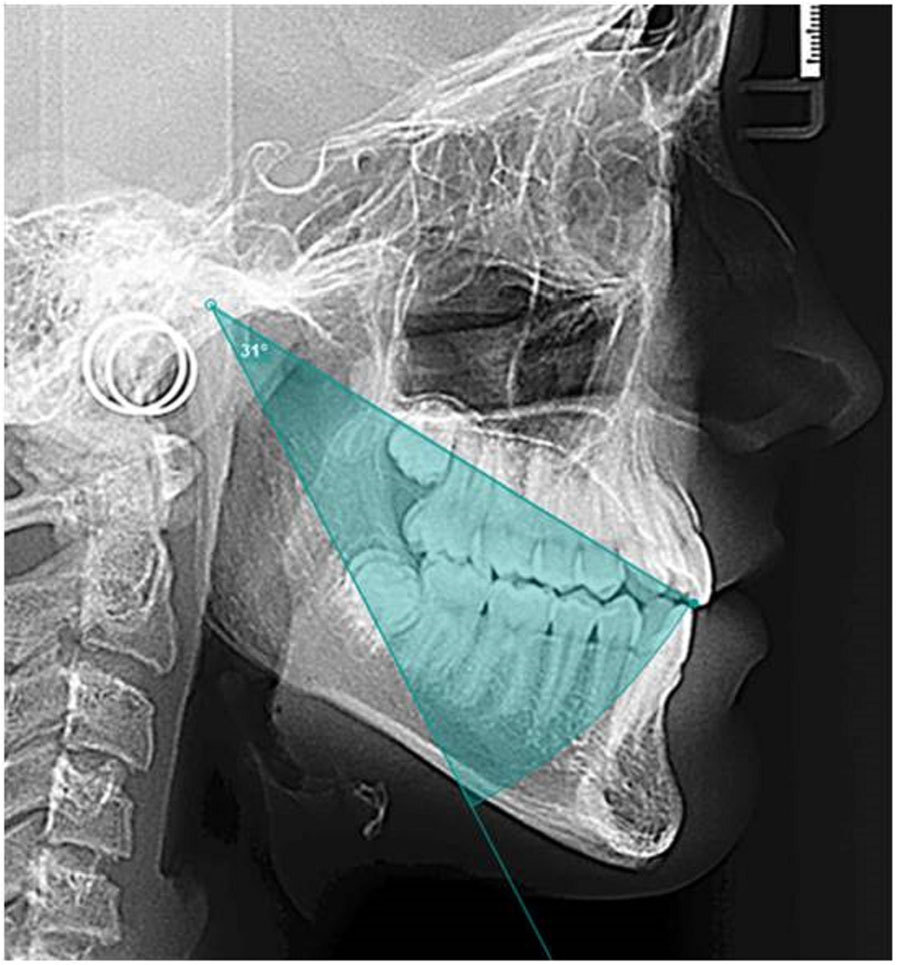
Rotational movement of the mandible. Shows how the rotational movement of the mandible was calculated in each patient with the cephalometric radiography. The rotational movement is an arch with its center in the condyle (landmark Co) and having the curve pass through the inferior incisor through the line connecting the condyle and inferior incisor (Co-II).
